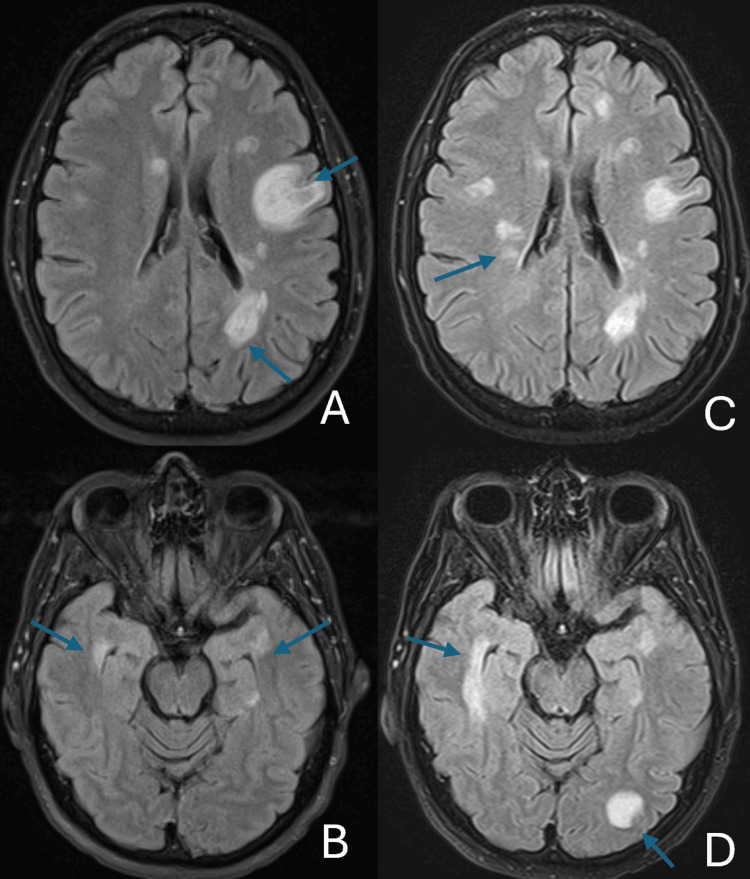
MRI of brain axial FLAIR-FS from the dates 3/1/21 (A, B) vs. 7/7/21 (C, D) showing progression of multiple lesions (arrows), subcortical and periventricular in distribution FLAIR-FS, fluid-attenuated inversion recovery with fat suppression
